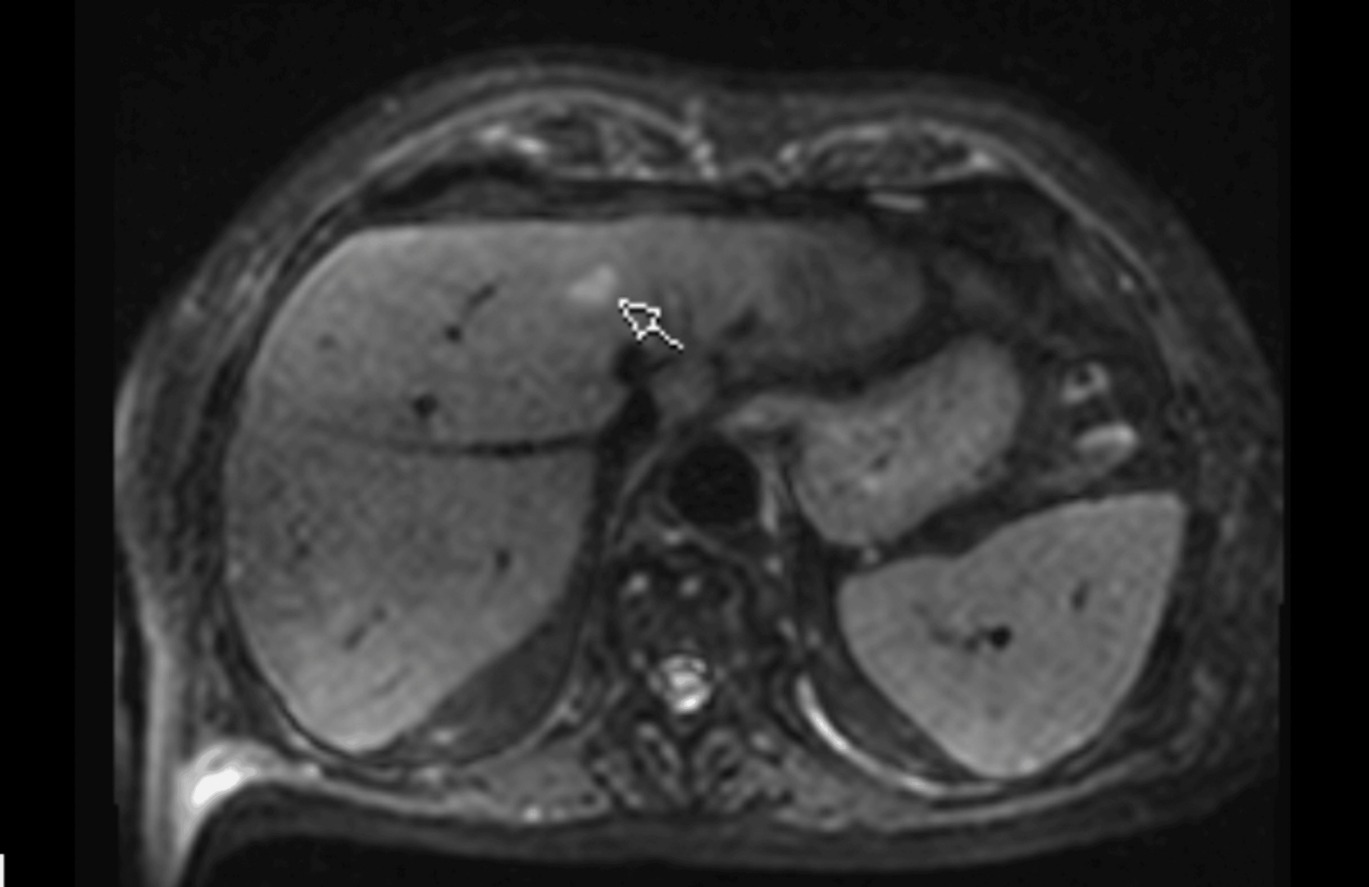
Magnetic resonance imaging (MRI) of the liver The arrow points to one of several hyperintense foci in the liver.
